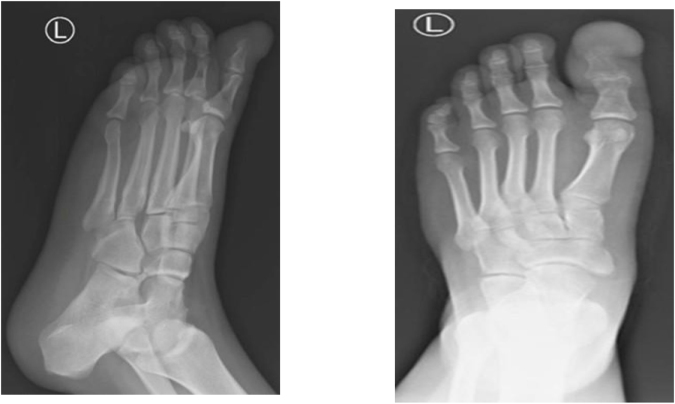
Sagittal MRI of the left foot revealing a heterogeneously enhancing soft tissue mass centered on the distal phalanx of the first toe.
